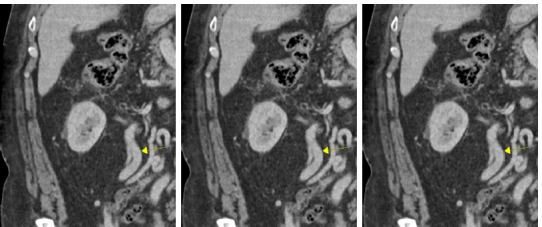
Right renal venous drainage 2 months status post nephrectomy. Coronal computed tomographic angiography of the abdomen and pelvis taken approximately 2 months post nephrectomy demonstrating right renal venous drainage through patent reno-gonadal anastomosis. Note that the yellow arrow in the figure refers to right renal venous drainage postoperatively.
